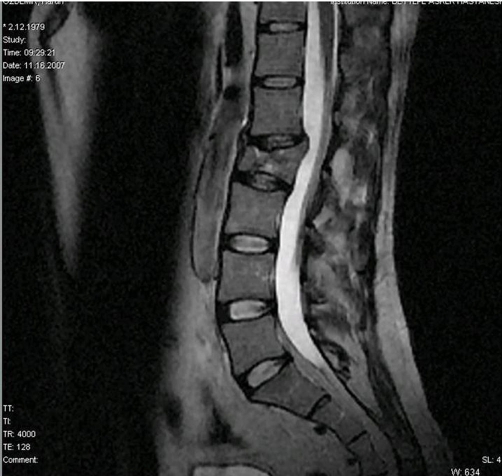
Sagittal MRI imaging shows mild compression of the spine cord.
